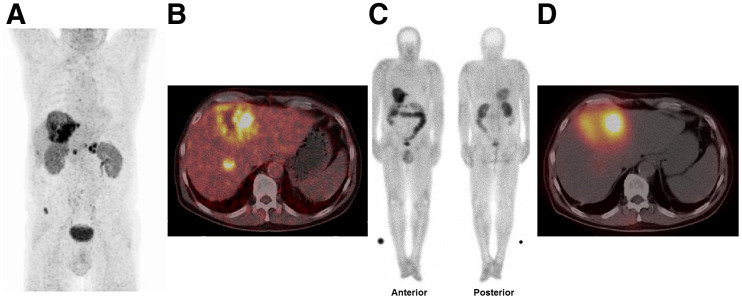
(A and B) Patient 4 had adenocarcinoma of pancreatic body, as well as hepatic, peripancreatic lymph node, and osseous metastases, which demonstrated high FAP expression on maximum-intensity-projection ^68^Ga-FAP-2286 PET image (A) and transverse ^68^Ga-FAP-2286 PET/CT image (B). (C and D) Significant uptake and late retention of ^177^Lu-FAP-2286 were noted in liver metastases on posttherapeutic whole-body scintigraphy in anterior and posterior views at 48 h after injection (C) and on transverse SPECT/CT image (D). Because of low resolution of ^177^Lu for imaging, as compared with ^68^Ga for PET/CT, not all tumor sites seen on ^68^Ga-FAP-2286 PET/CT are apparent on ^177^Lu-FAP-2286 images.
